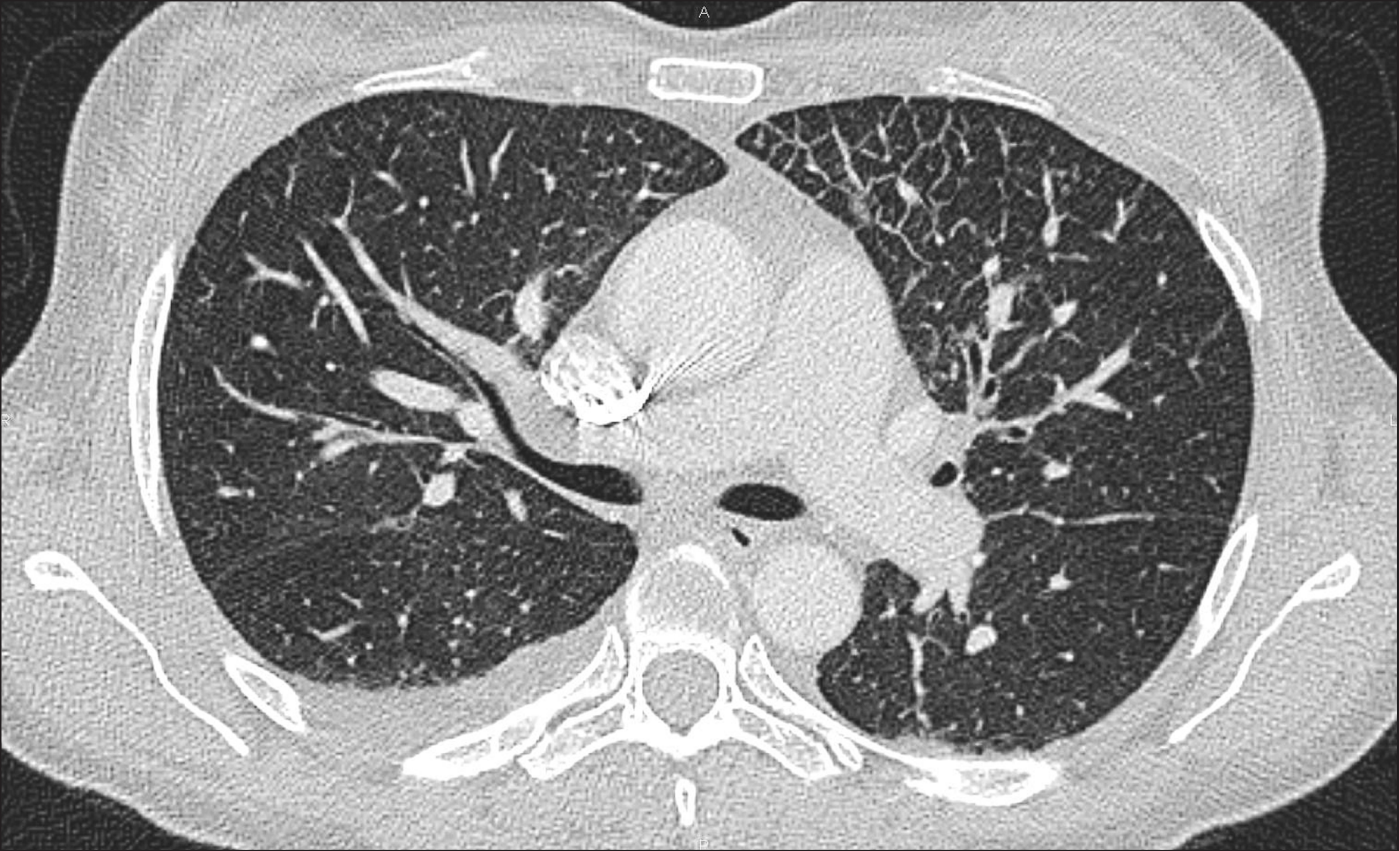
Chest HRCT showing interlobular septal thickening, with a prominent left anterior location, and small pleural effusion.
